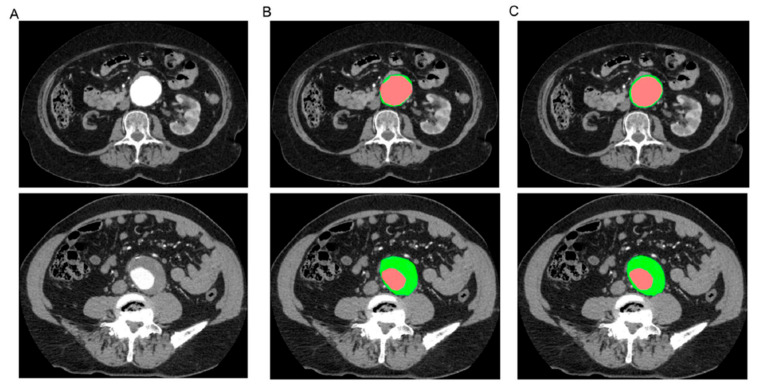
Representative images of the segmentation of the aortic lumen (in red) and the intraluminal thrombus (in green). (A) CT scan cross-sectional views of patients with infrarenal AAA. (B) Manual segmentation. (C) Automatic segmentation. Reprinted with permission under open access from Lareyre et al. [201].
